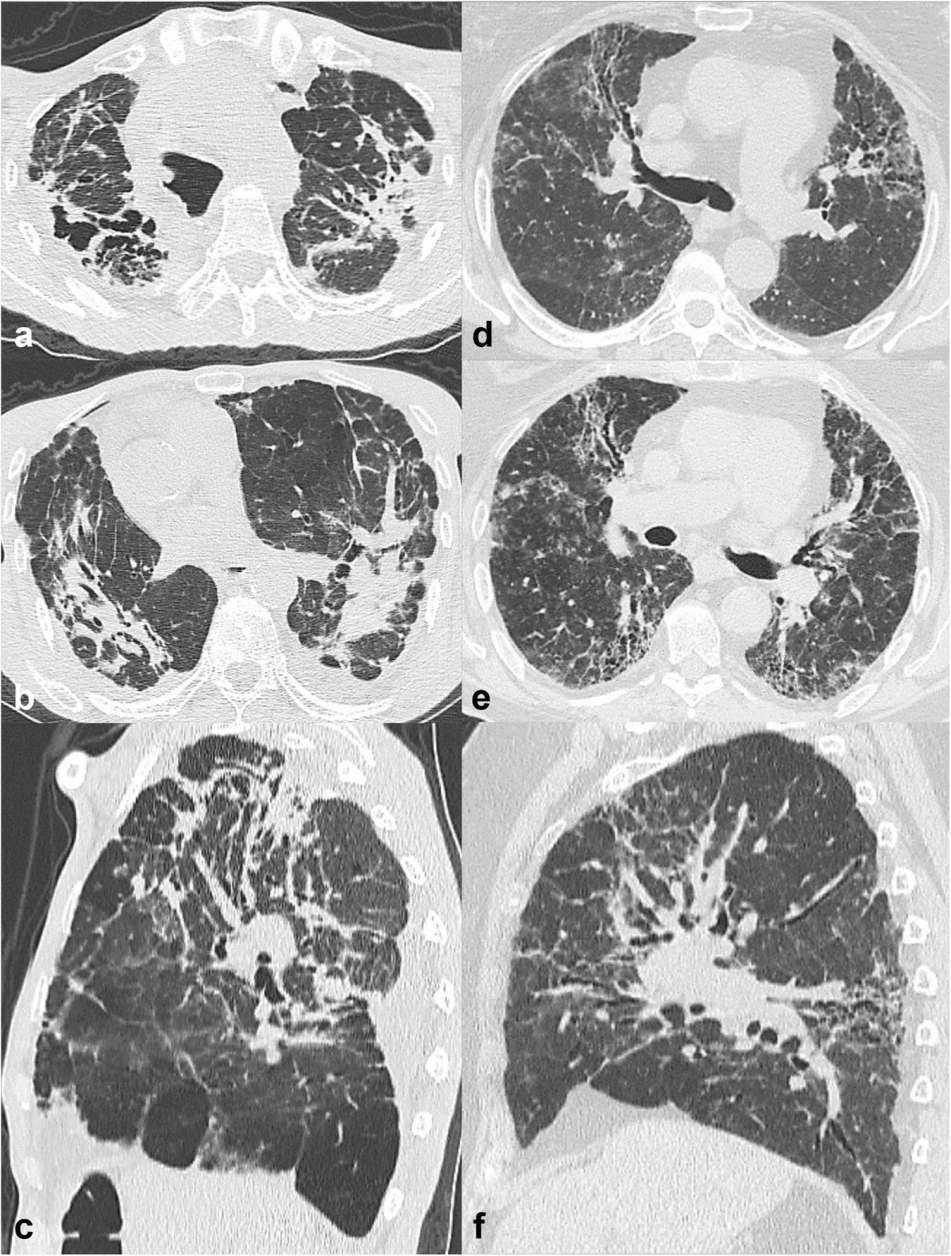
Computed tomography (CT) patterns of fibrotic lung diseases with upper-mid predominant distribution. Axial and sagittal CT images (a–c) showing peribronchovascular conglomerate masses in the upper lobes associated with parenchymal and airways distortion in the right upper lobe in a fibrotic sarcoidosis. Note the right upper lobe volume reduction and areas of mosaic attenuation in the mid-lower zones due to air trapping. Axial and sagittal CT images (d–f) demonstrating reticulation and mild traction bronchiectasis with a peribronchovascular predominant distribution, in a fibrotic hypersensitivity pneumonitis. Note the association with centrilobular ground-glass nodules, partially confluent, and a mild ‘three-density pattern’ in the upper-mid zones
